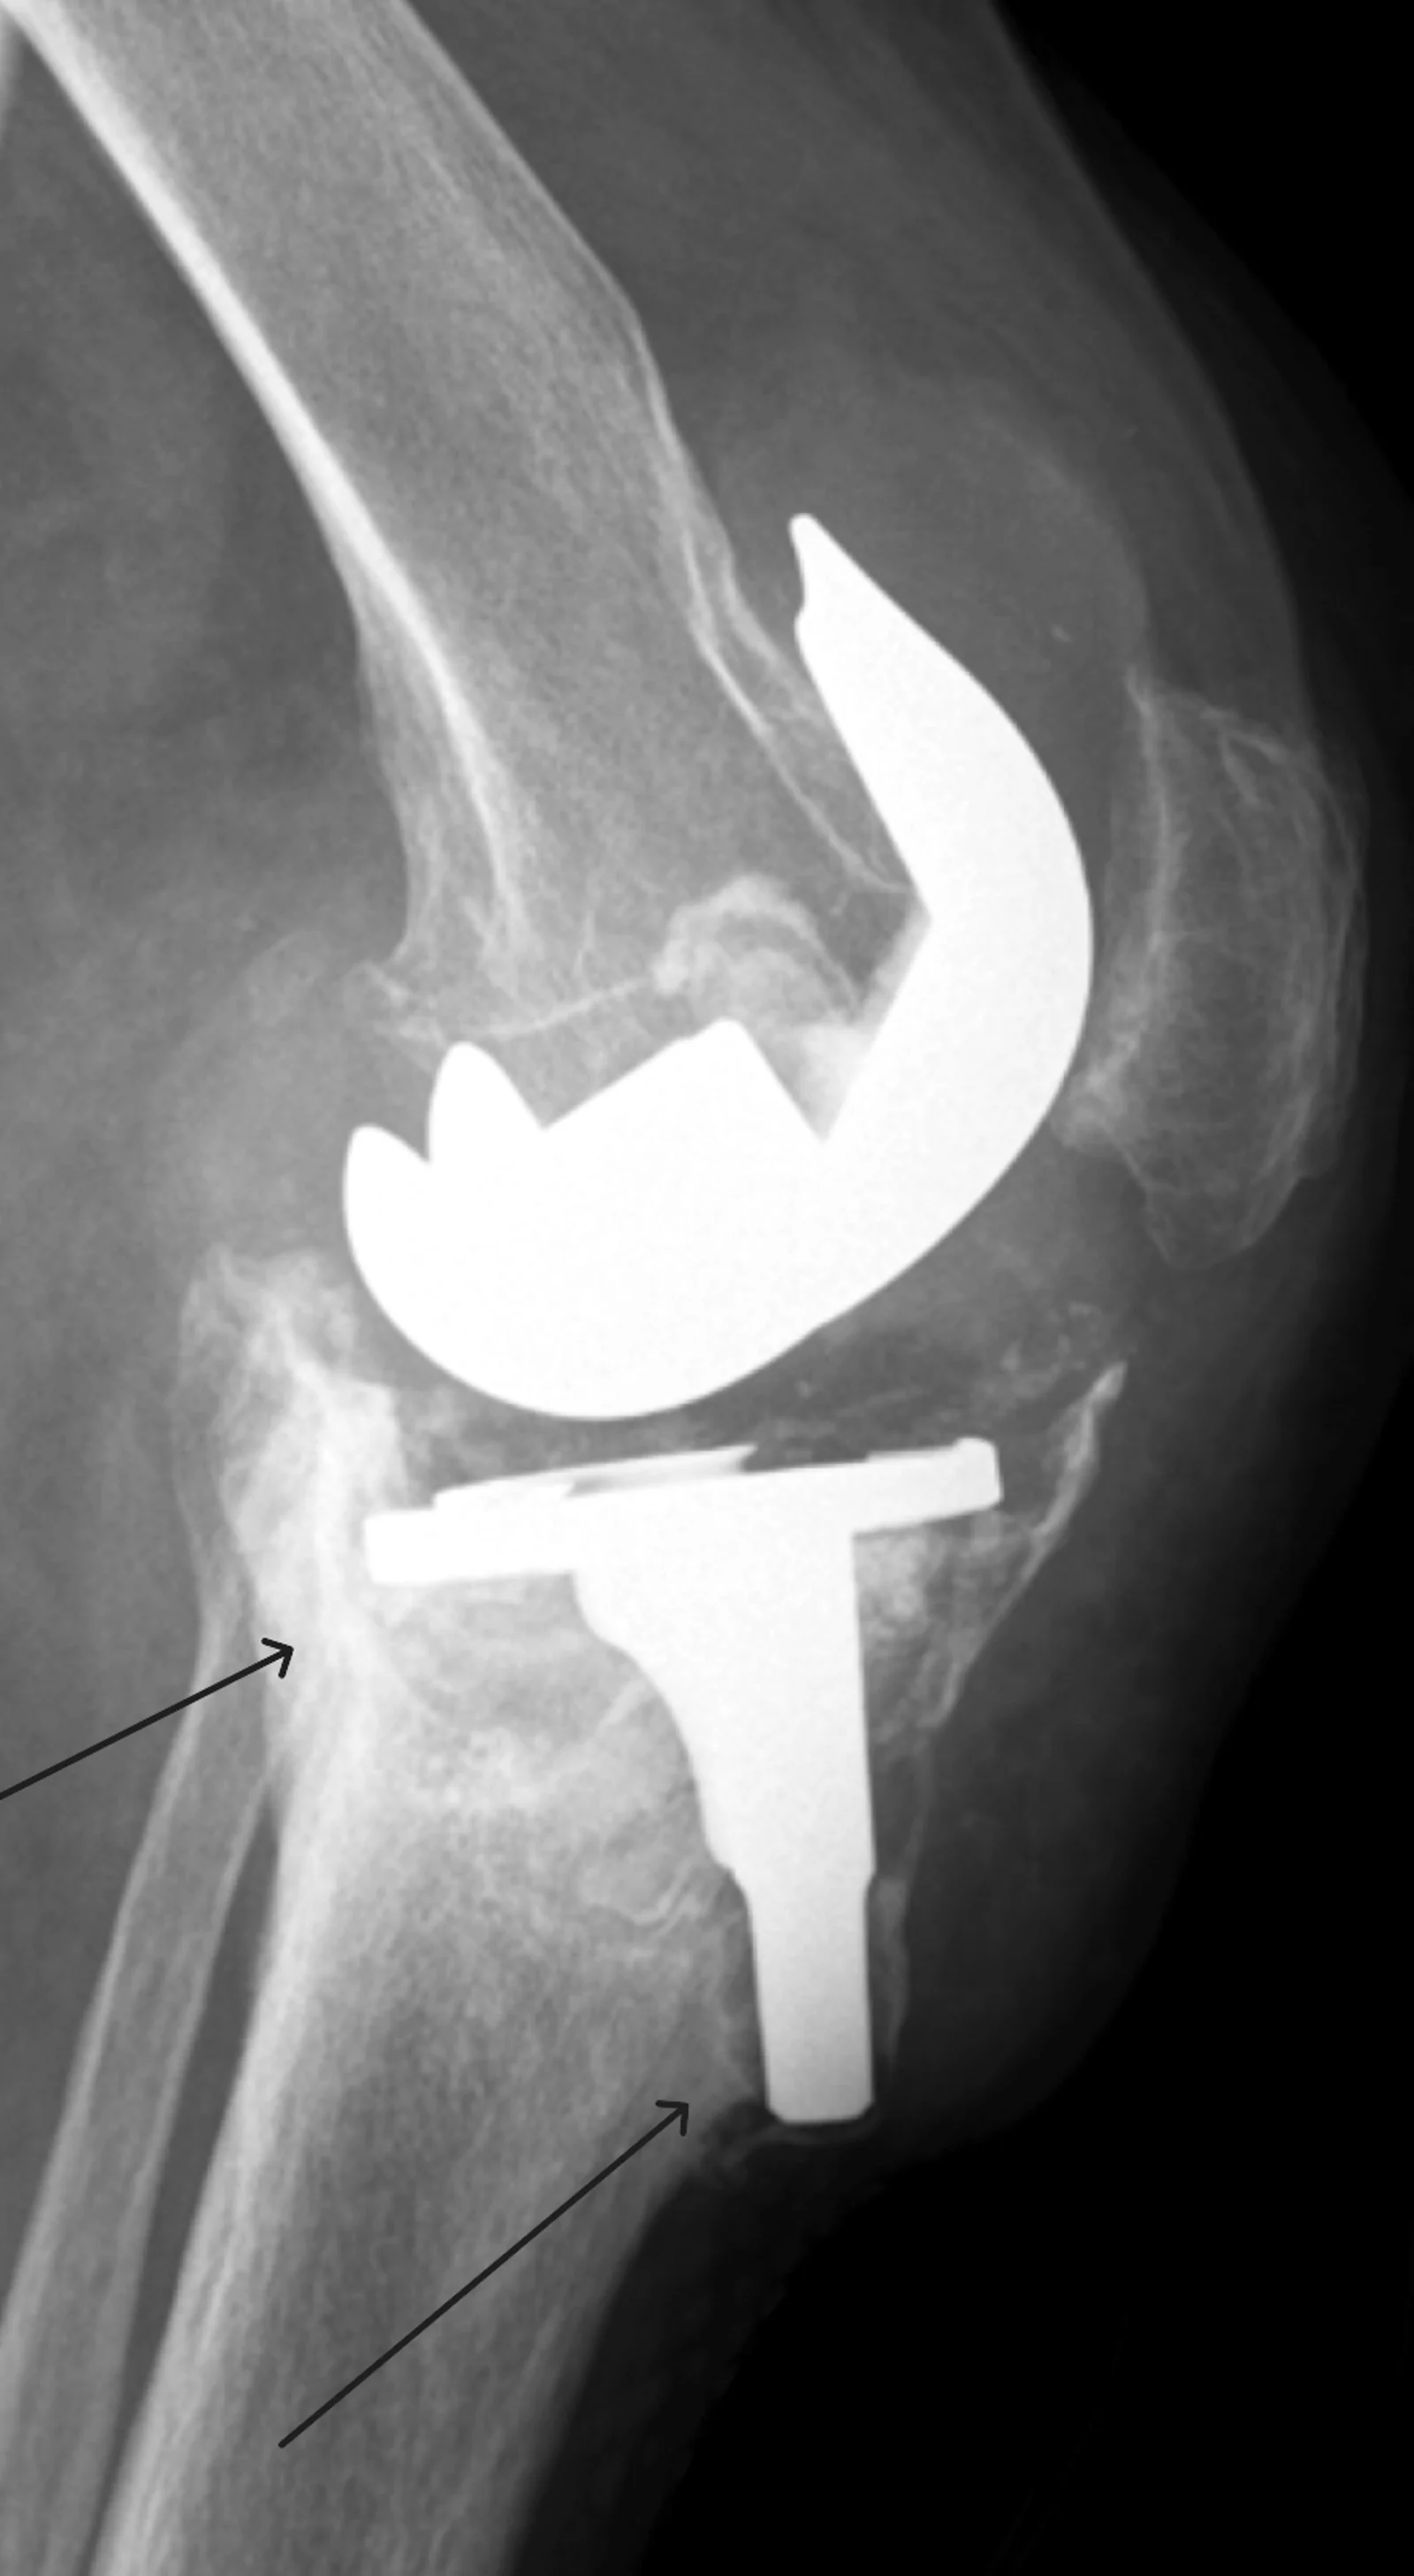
Lateral radiograph of the left knee showing osteolysis of the proximal tibia approximately nine years after primary TKA. TKA, total knee arthroplasty
